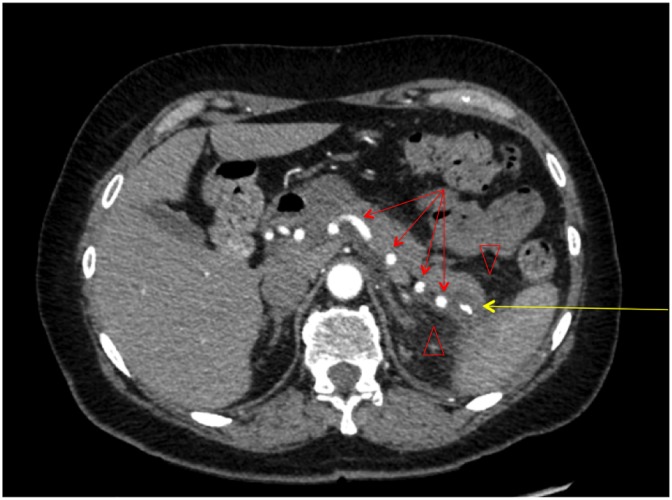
Computed tomography of the abdomen (axial view) displaying a calcified, cystic mass in the tail of the pancreas with an area of central scarring (yellow arrow) and evidence of peripancreatic inflammation and edema (red arrow heads). The splenic artery can be seen coursing through the pancreas in a tortuous manner (red arrows).
